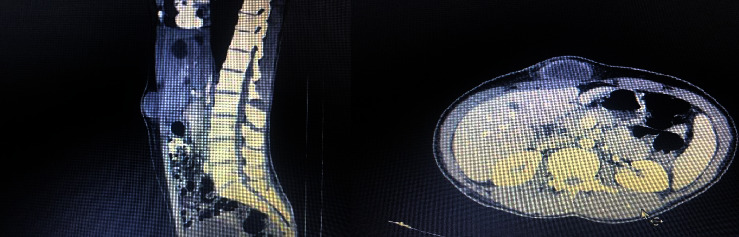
Abdominal CT scan with contrast shows a cystic lesion with wall thickness internal echogenicity and fat stranding without enhancement.
